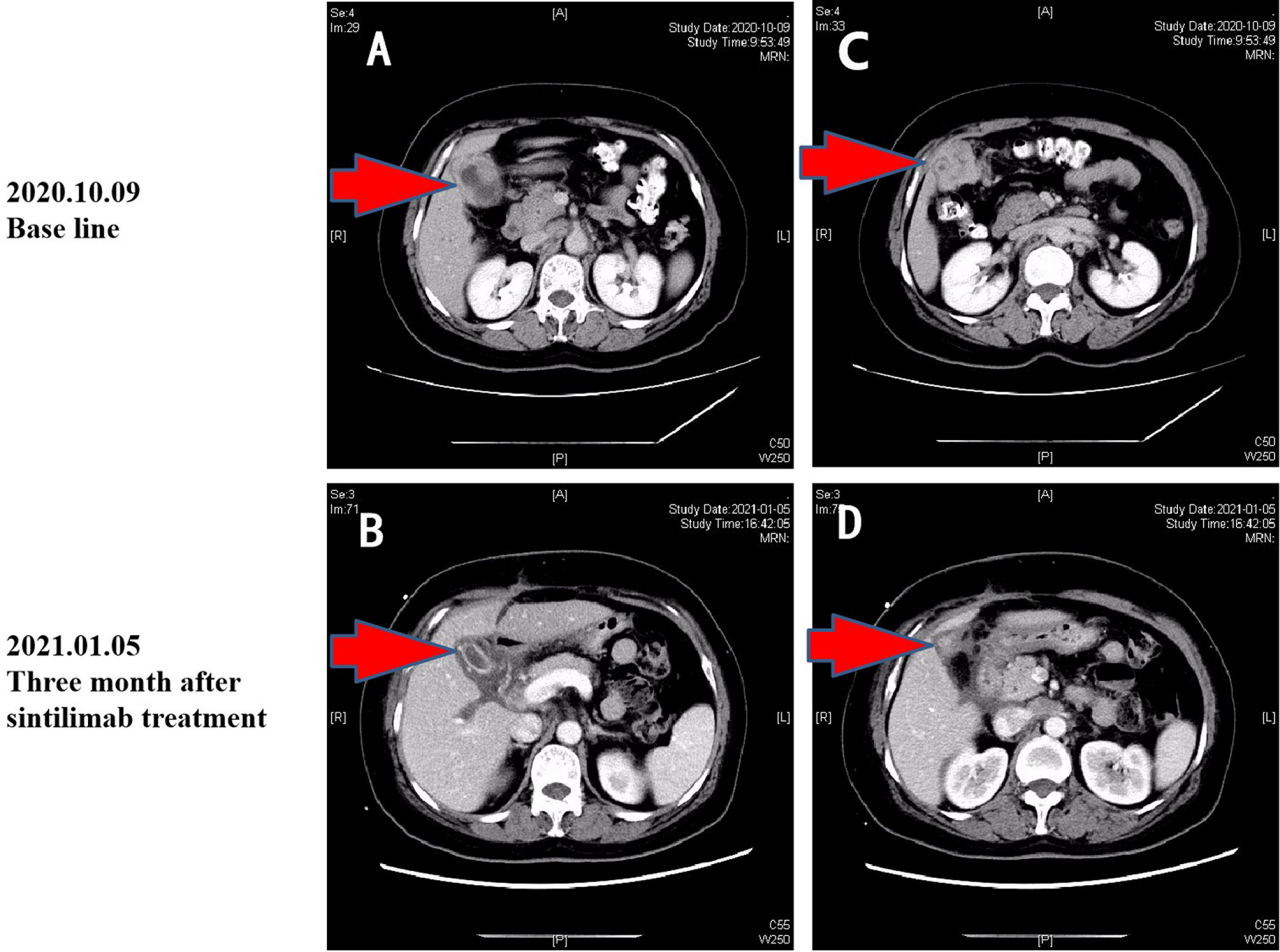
CT images of baseline (A, C) and after sintilimab treatment (B, D).
